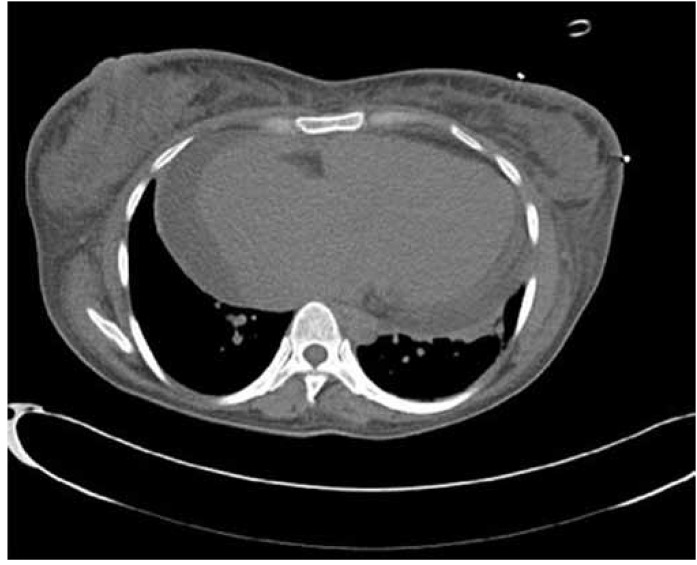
Non-enhanced CT scan of the chest.
